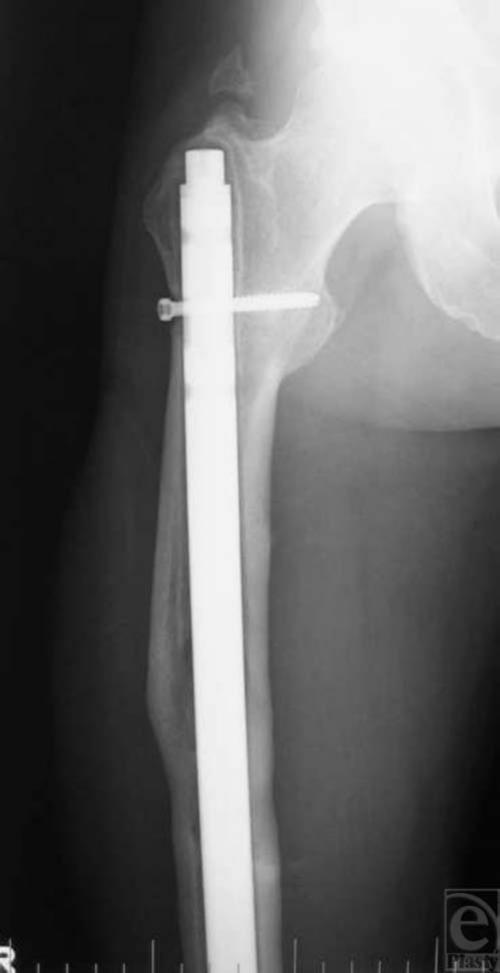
Radiograph showing final healing of the femoral shaft fracture.
